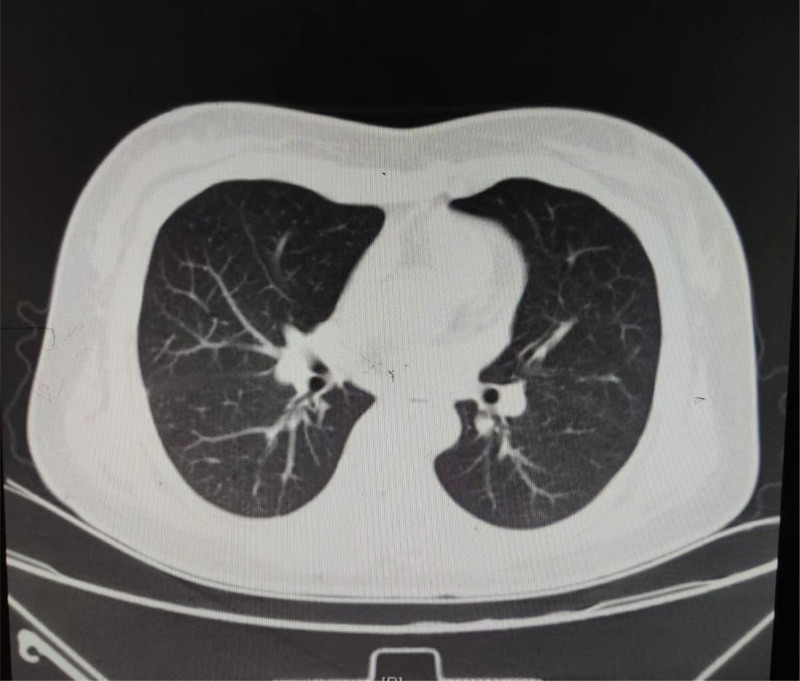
The chest CT of patient.
